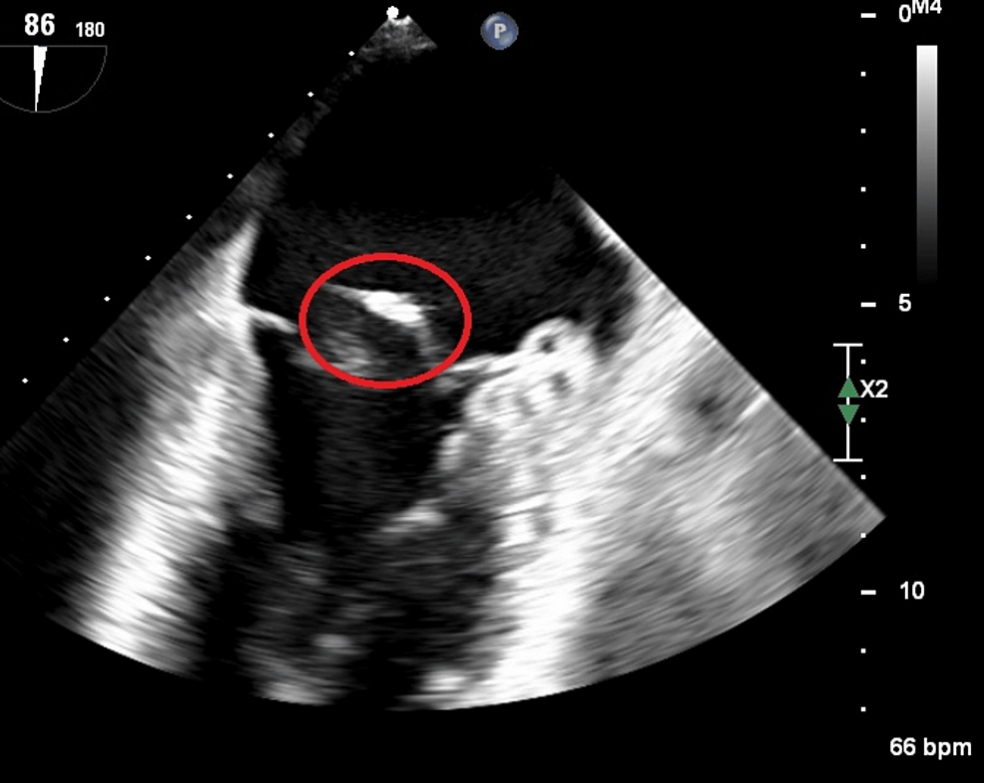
Transoesophageal echocardiographic image showing vegetation on mitral valve leaflet. The red circle demarcates vegetation.
